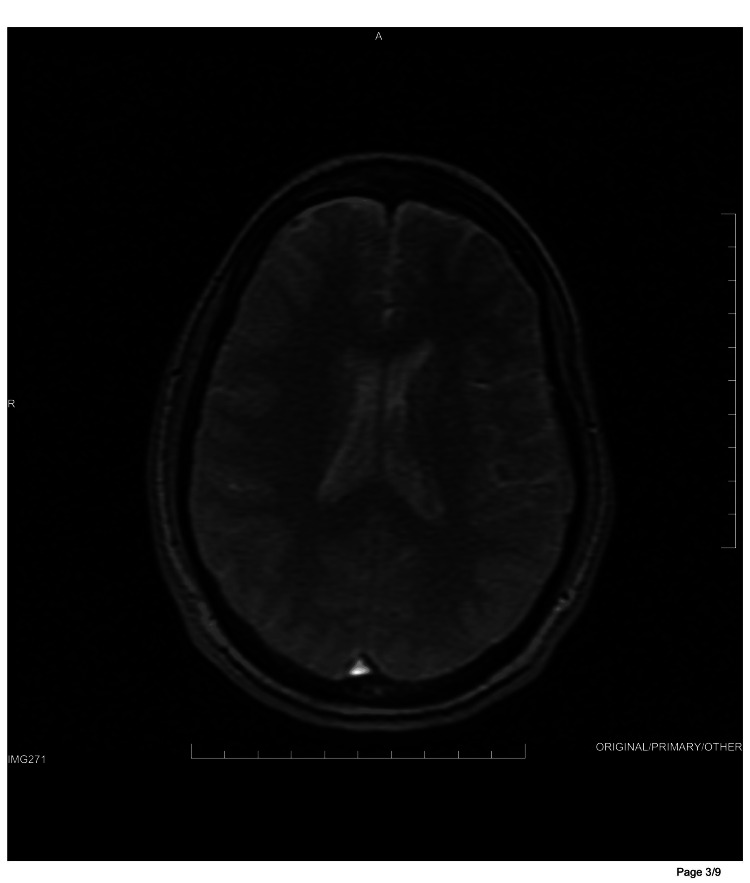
MRI showed no acute infarction or hemorrhage No diffuse brain atrophy in the prefrontal cortex and basal ganglia, no diffuse white matter lesions affecting the frontal and temporal lobes, and no alterations in the caudate nucleus and putamen
